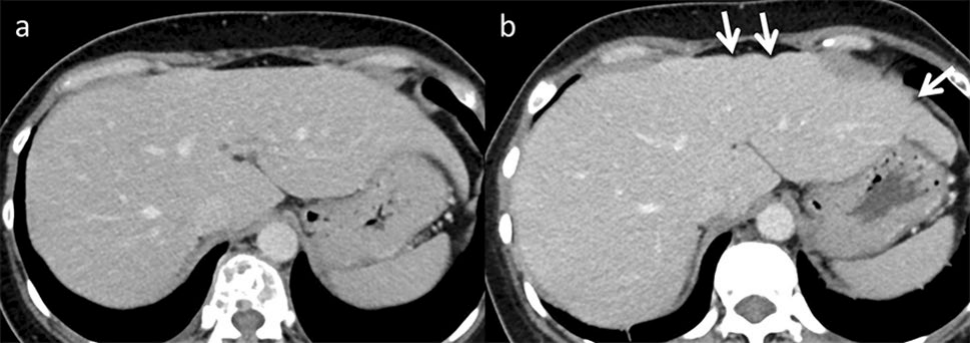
Pseudocirrhosis in an asymptomatic 39-year-old woman, who was undergoing chemotherapy (CTX, paclitaxel, and bevacizumab) for left-sided breast cancer. a Contrast-enhanced computed tomography (CT) reveals a smooth hepatic margin before CTX. b A nodular contour is observed in the lateral liver segment on contrast-enhanced CT performed 7 months after the initial CTX. It is difficult to differentiate pseudocirrhosis from liver cirrhosis on a single image alone
